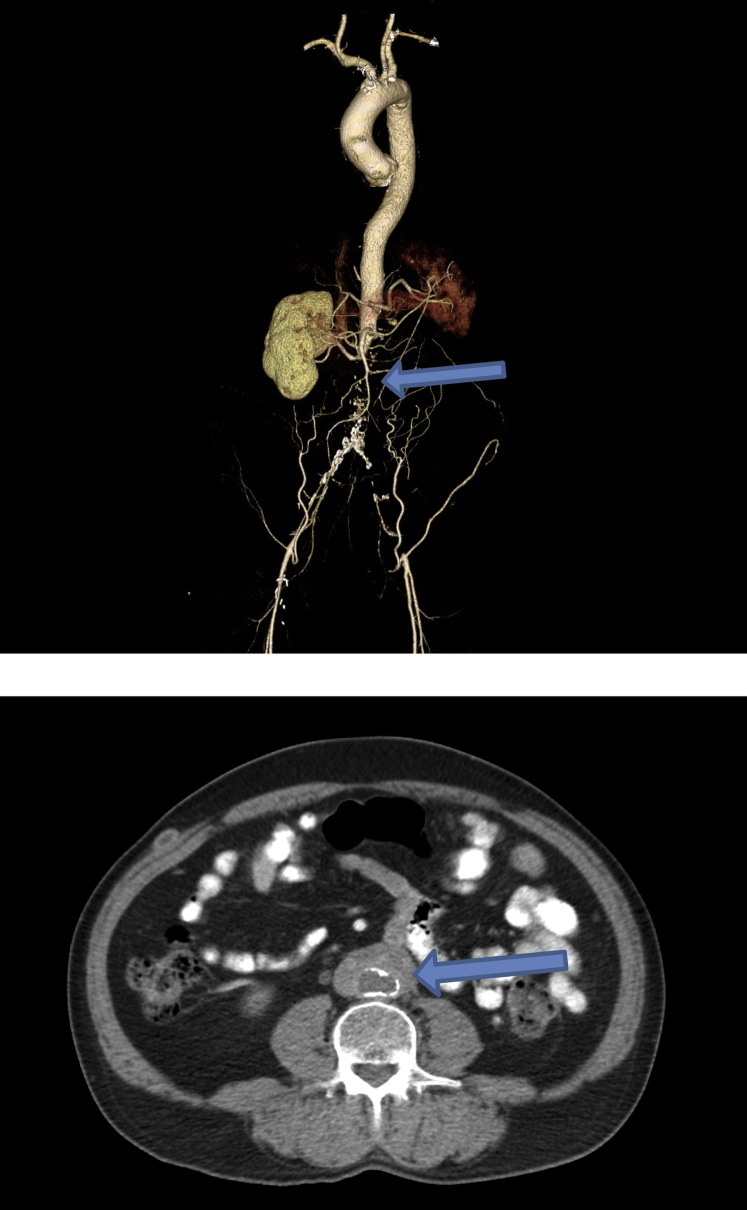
Computed tomographic angiography (CTA) in a 65-year-old man with retroperitoneal fibrosis (RBF) and aortic occlusion. The top panel shows a 3D reconstruction of the aortoiliac occlusion (*arrow*); note the absence of dye in the axillobifemoral bypass graft, which is also occluded. The bottom panel shows the occluded infrarenal aorta in cross-section incorporated within a dense, periaortic mass typical of RBF (*arrow*).
